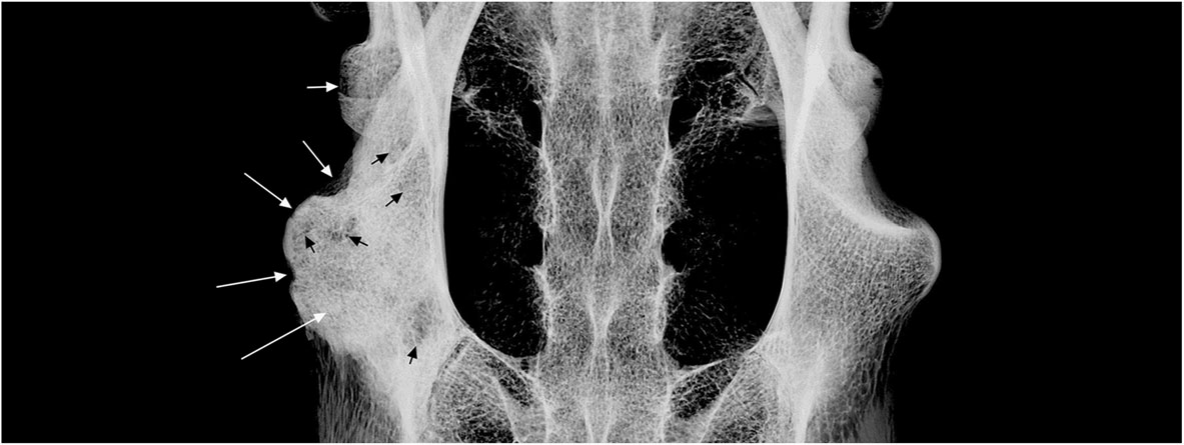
Radiograph of the right acetabulum of *Tamandua tetradactyla* (NPM 069). White arrows, bone remodeling; Black arrows, radiolucent regions indicating bone rarefaction
